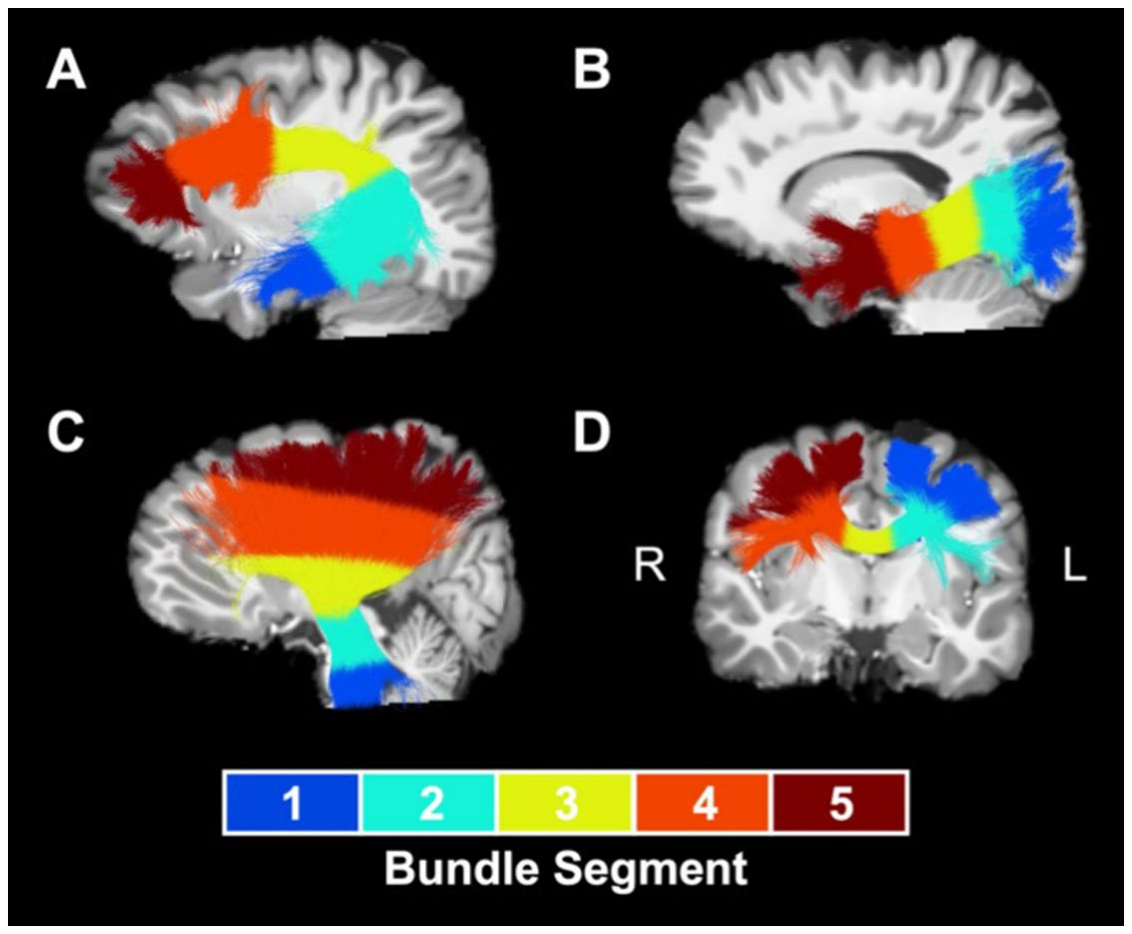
Segment numbering convention for sample bundles. Numbered segments for a representative collection of white matter bundles: (A) left arcuate fasciculus, (B) left inferior longitudinal fasciculus, (C) left corona radiata, and (D) rostral body of the corpus callosum. Bundles are overland on a T1-weighted anatomical reference image. L, left; R, right.
